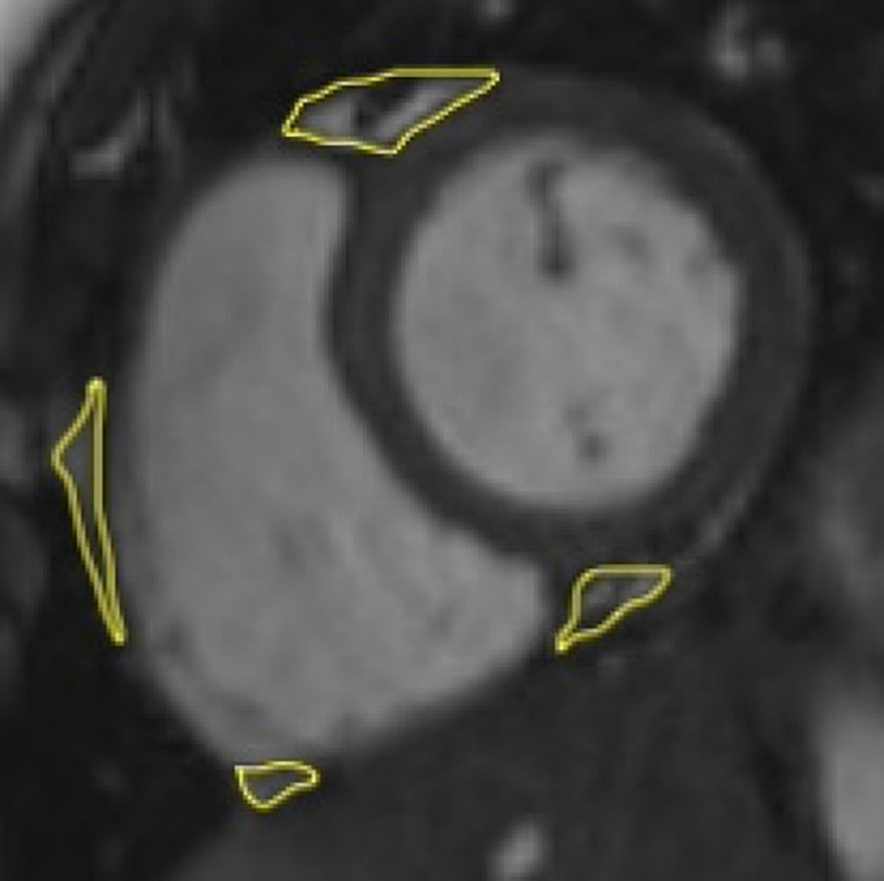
Cine segmented steady-state free-precession MRI of the heart in 14-years-old boy diagnosed with arterial hypertension. Contours of epicardial adipose tissue (EAT) are outlined by hand on end diastolic short-axis image. The EAT volume (EATV) is 14.89 cm^3^.
